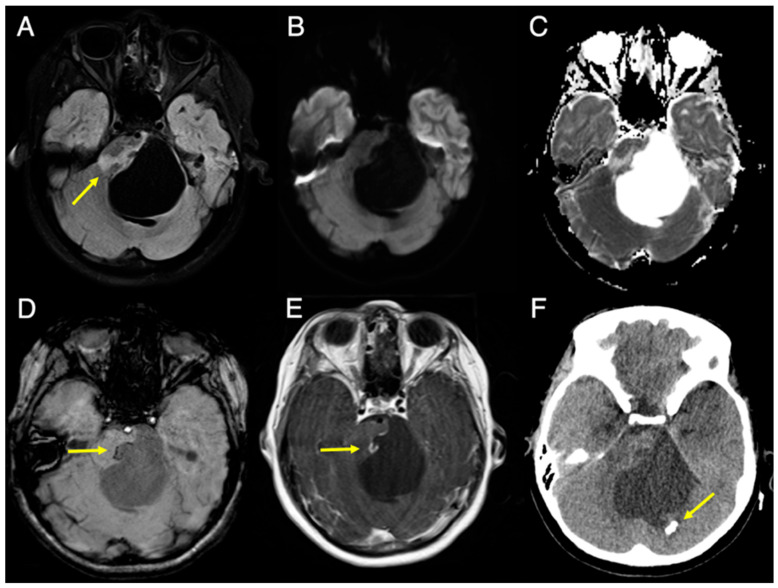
Axial 3T MRI and CT slices of the cystic lesion at the level of the pons. (A) Fat-saturated FLAIR image shows patchy T2 hyperintensity in the adjacent pons (arrow). (B) DWI and (C) ADC map shows facilitated diffusion inside the cyst. (D) SWI shows linear focus of susceptibility, possibly representing microhemorrhage or calcification in the cyst/pontine parenchyma junction (arrow). (E) Contrast-enhanced T1-TSE shows corresponding linear enhancement (arrow). (F) Same-day CT image (brain window) shows a coarse calcification in the posterior cyst wall (arrow).
